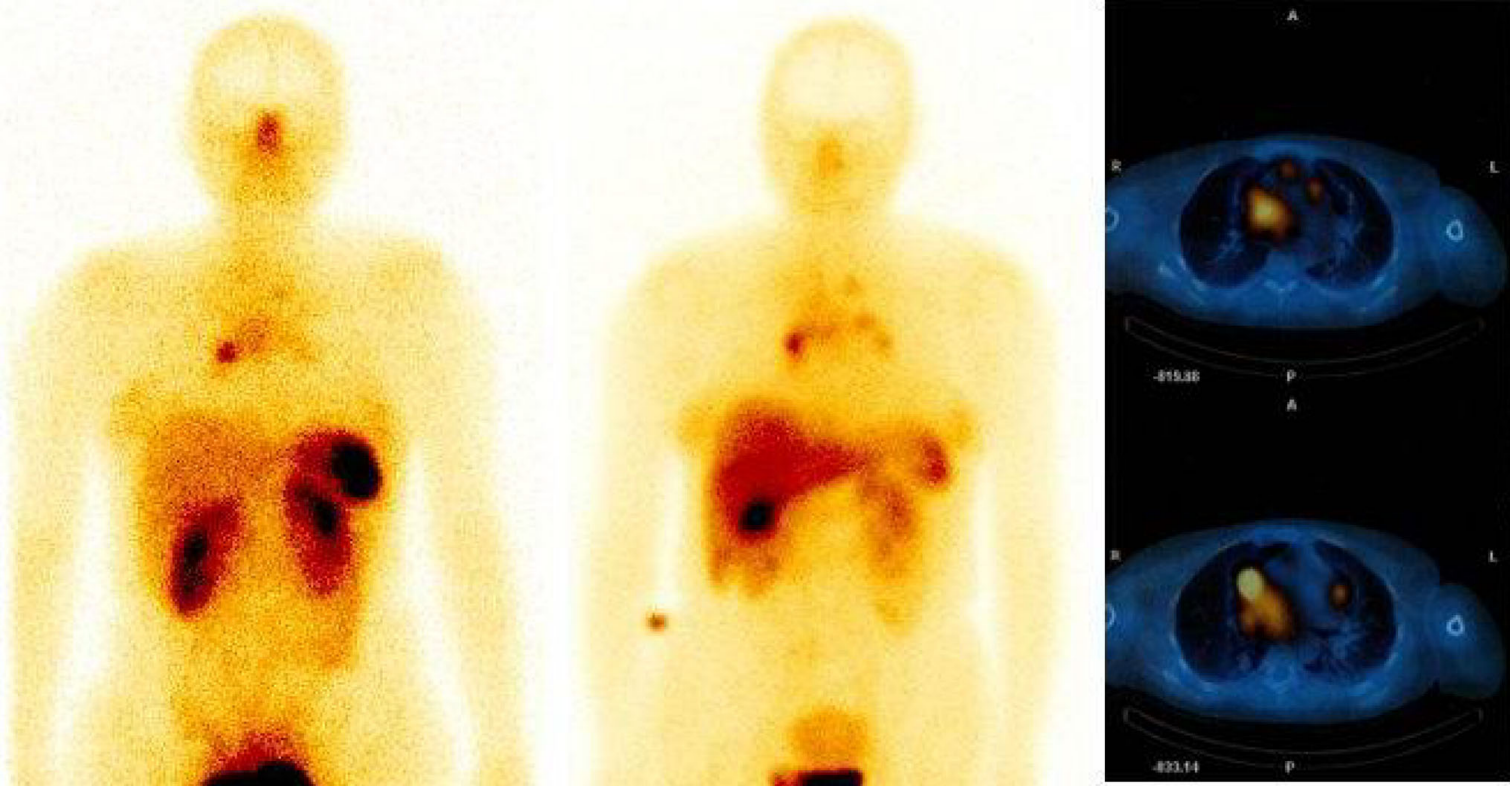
Peptide receptor scintigraphy using ^99m^Tc-HYNIC-TOC in a patient with mediastinal lymph node metastases of differentiated thyroid carcinoma (planar and SPECT/CT images)
